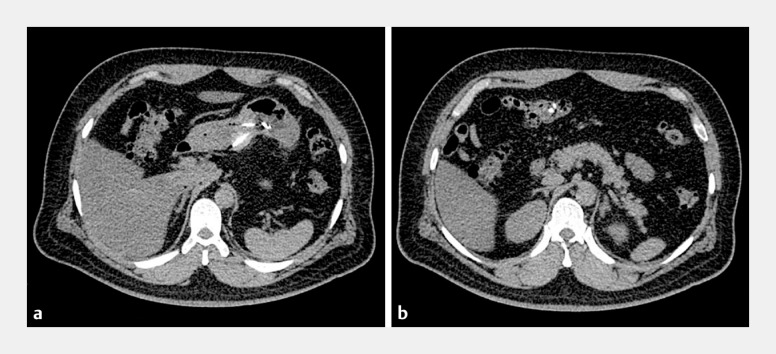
Cervical thoracoabdominal computed tomography images showing several high density shadows:
